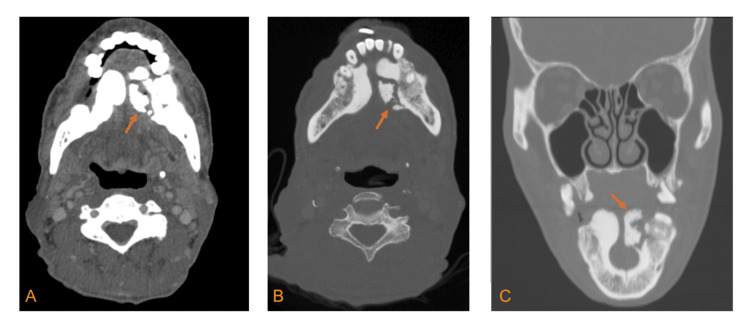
CT images showing bilateral mandibular tori with the left torus showing osteolytic changes A) axial soft tissue window view B) axial bone window view C) coronal bone window view In Figures 1A-1C, the arrows highlight the torus mandibularis with the region of osteonecrosis/osteolysis. No direct communication with periodontal/periapical lucency or a radicular cyst related to any of the adjacent teeth was identified. Mild surrounding fat stranding was present without overt swelling, fluid collection, or disruption of the fat planes. Mild reactive lymphadenopathy in the left submandibular region was noted.
